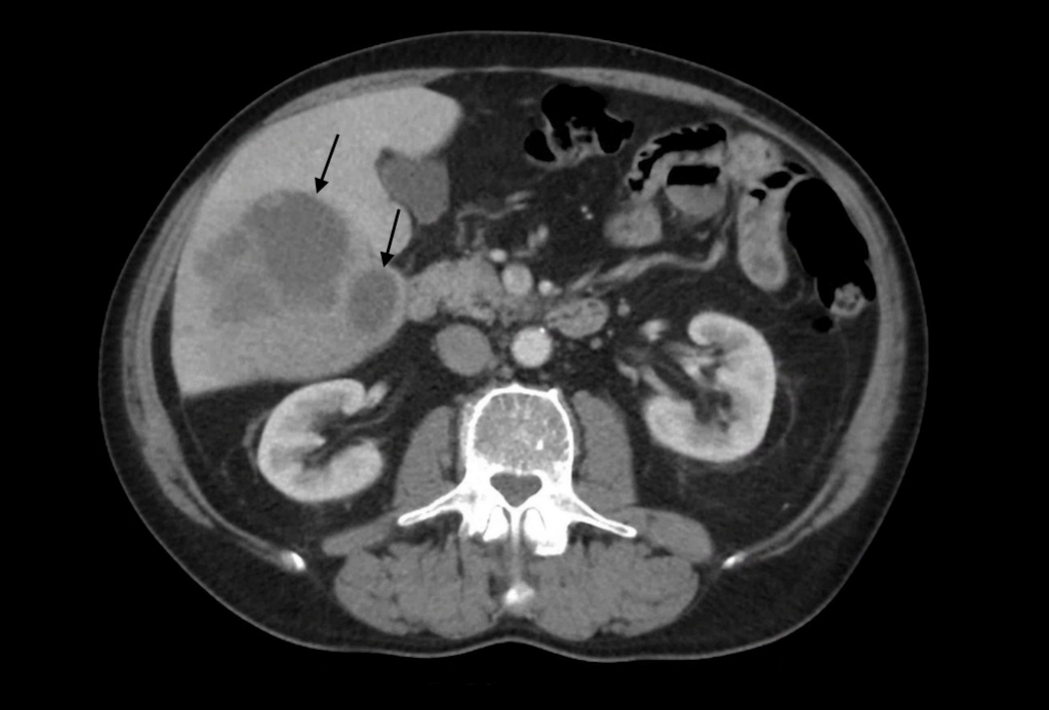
Contrast Enhanced Tomography of the Abdomen Contrast enhanced computed tomography of the abdomen performed on the day of presentation demonstrates focal areas of hypoattenuation within the right hepatic lobe (arrows) with subtle adjacent hypoattenuation predominantly in segment 6. Note the lack of perilesional hyperenhancement.
